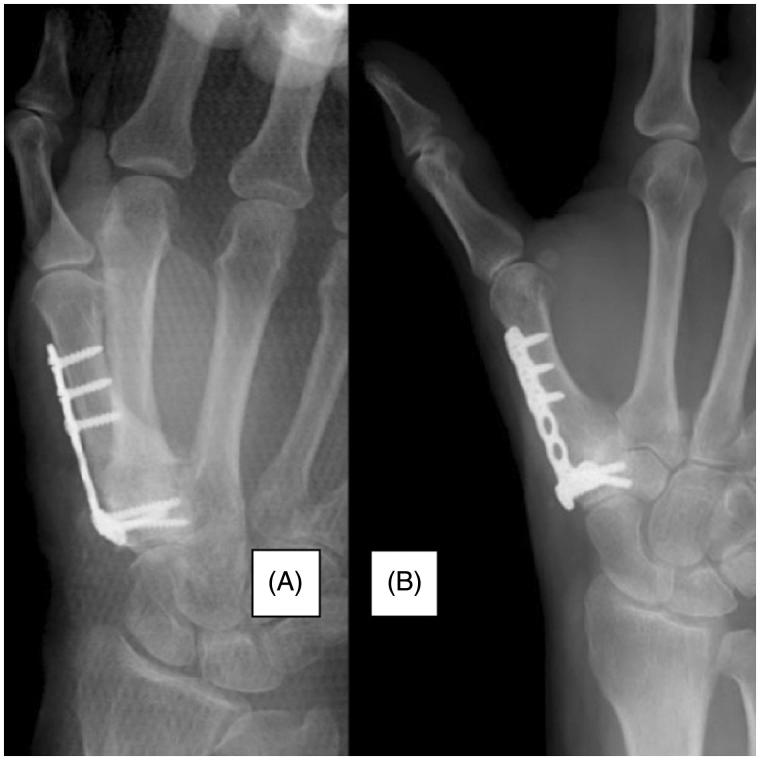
Case 1: a 58-year-old female. (A) Immediately postoperative radiograph of the right hand (dorsoplantar view) after the second surgery. (B) At 2 months after the second surgery, radiography confirmed bony union.
